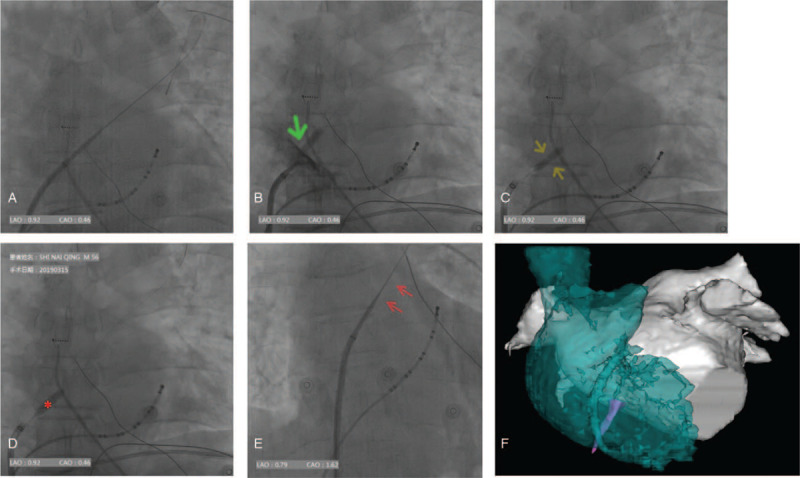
Process of modified ABS. Swartz sheath failed to pass interatrial septum with routine TSP procedure. Coronary angioplasty balloon inflated and contrast injected. The green arrow indicates the “Hitting Wall” sign--the position of interatrial septum. The guidewire has been switched to soft head BMW and dilator of the sheath was removed. Balloon atrial septoplasty. The “waist” of inflated balloon (yellow arrows) represented the interatrial septum. The balloon was reinflated several times and the “waist” disappeared (red asterisk) indicating a successful ABS. TSP sheath assembly passes through interatrial septum after septoplasty via 0.032” J-tip guidewire (red arrows). Merged images from cardiac CT reconstruction and CARTO FAM. The magenta structure represents the position of angioplasty balloon and the route of TSP. ABS = atrial balloon septoplasty, TSP = transseptal puncture, FAM = fast anatomic mapping.
